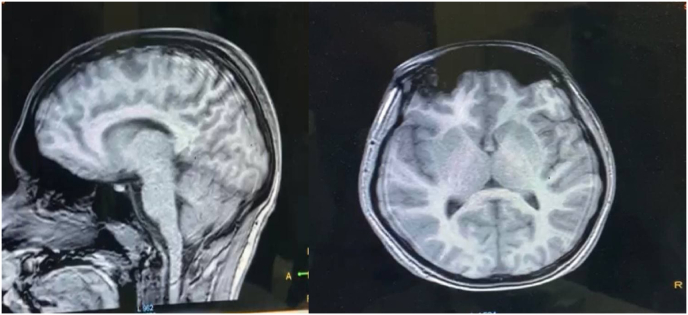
[a, b]: MRI revealed normal finding at First time(1T).
